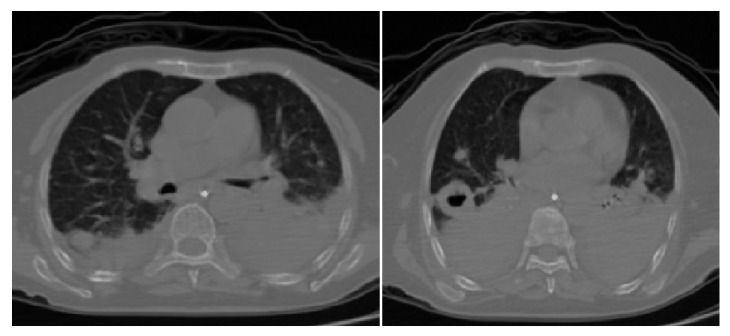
Parenchymal window of computed tomography scan showing bilateral pleural masses, effusions, and enlarged cavitary lesion at the time of respiratory insufficiency.
